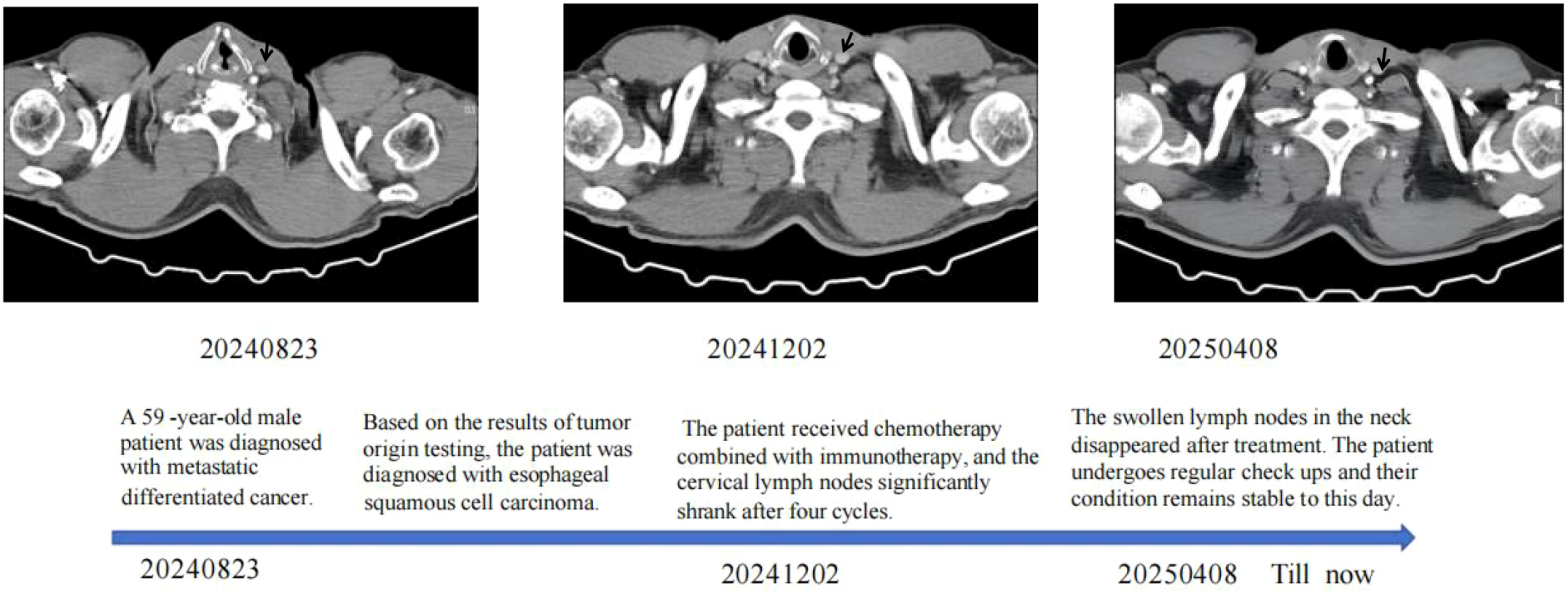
The patient's (case 2) clinical course schedule. Computed tomography scans of chest lesions before treatment (20240823), under treatment (20241202), and after treatment (2025 04 08). The patient got the treatment with immunotherapy (Tislelizumab/Adebrelimab) combined with chemotherapy (nab-paclitaxel and carboplatin). The patient undergoes regular follow-up examinations and has achieved a PFS of 12 months so far.
